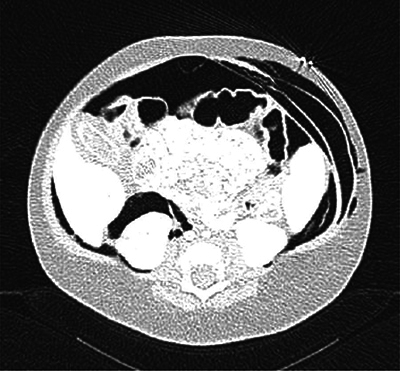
Whole body low-dose CT scanner. Cleavage of all abdominal layers produced by the free air.
